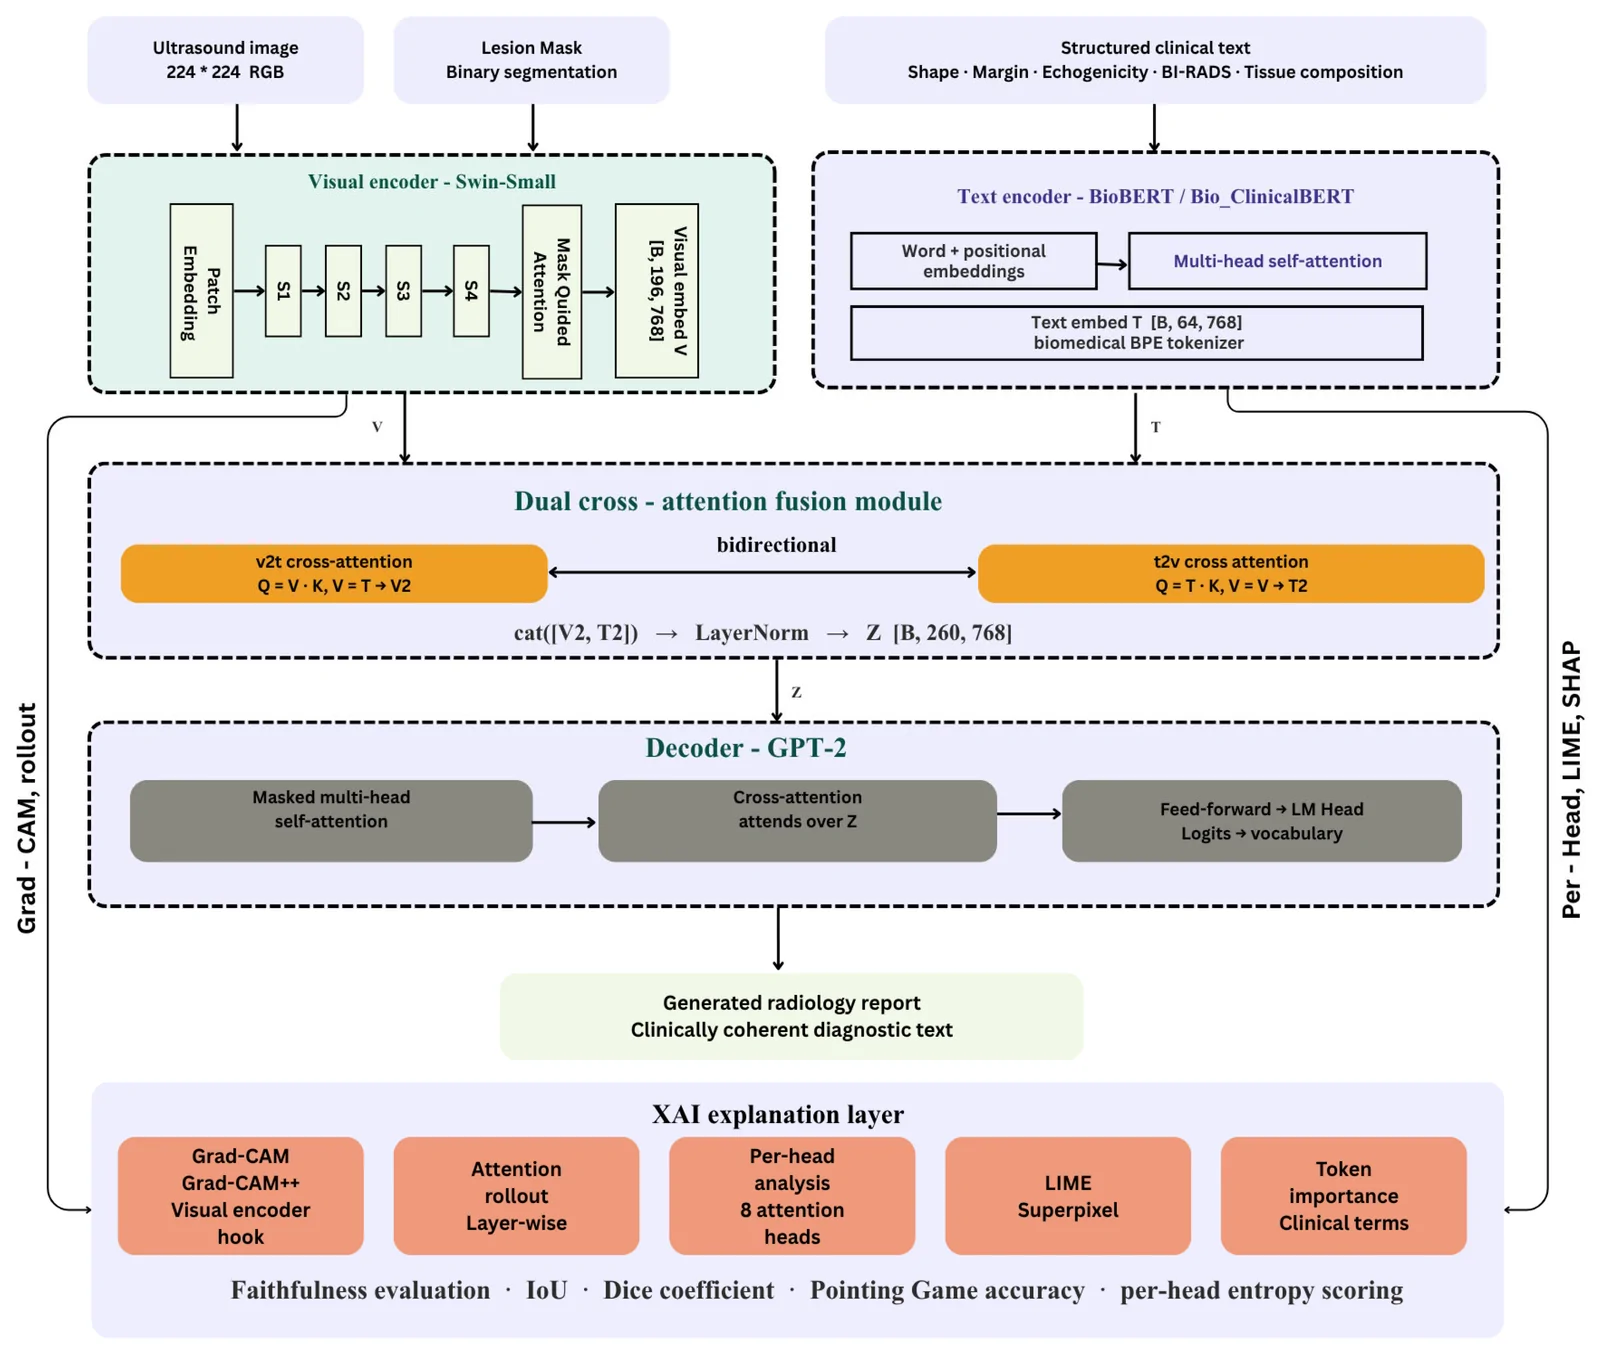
Explainable multimodal framework for breast ultrasound report generation.
